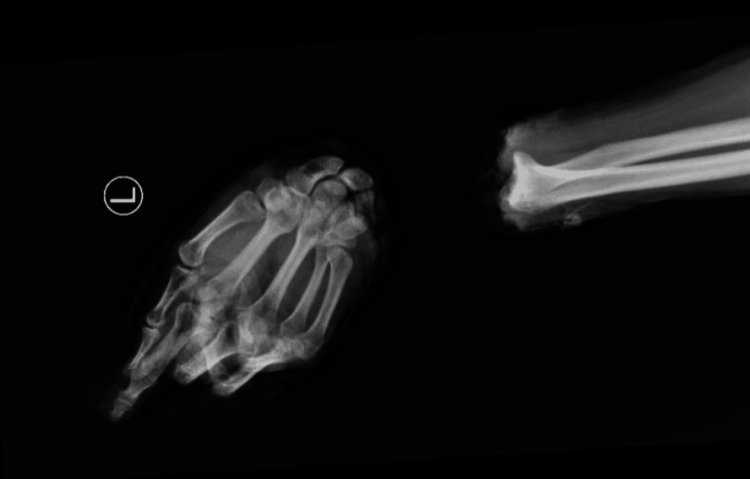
Initial radiographs demonstrating complete amputation through the radiocarpal joint
